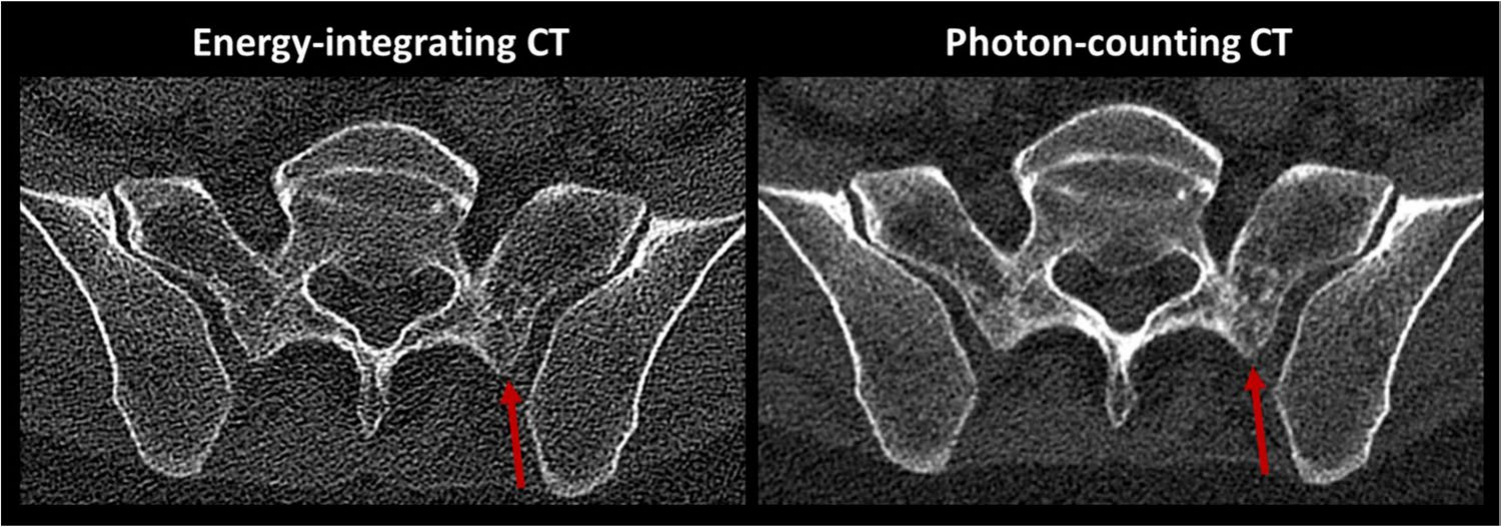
Exemplary image of a 44-year-old male patient. PC-CT shows superior delineations of the trabecular structure (red arrow) and a substantially lower image noise compared to EID-CT. Of note, the CTDI_vol_ of the EID-CT was 11.45 mGy vs. 5.56 mGy for the PC-CT examination. EID-CT = energy-integrating CT; PC-CT = photon-counting CT; CTDI_vol_ = volume computed tomography dose index
